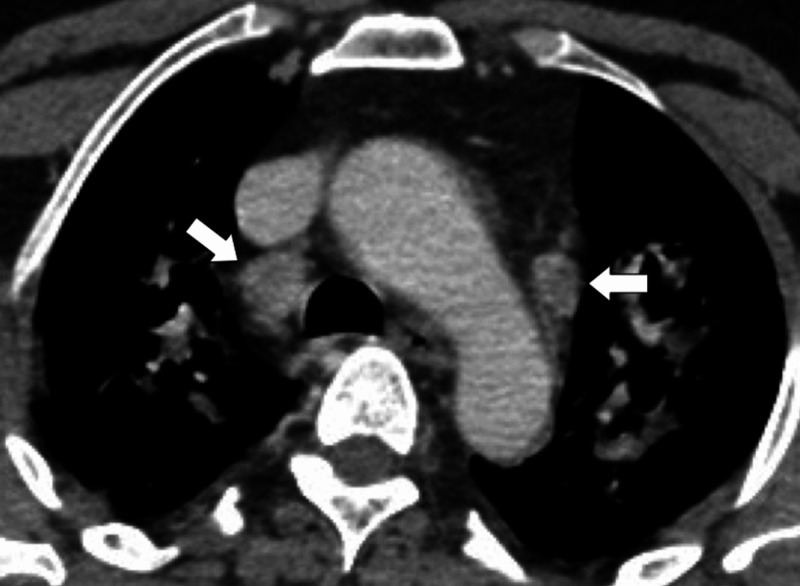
Patient 3 axial CT slice An axial computed tomography (CT) slice through the chest in the same patient, in mediastinal windows, demonstrates mediastinal lymphadenopathy (white arrows).
